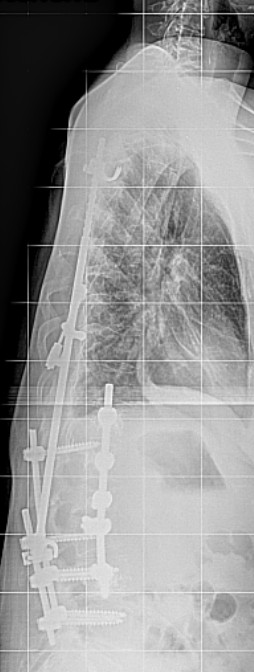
X-ray of the spine in lateral projection one year after additional ventral augmentation showing no further loss of correction
